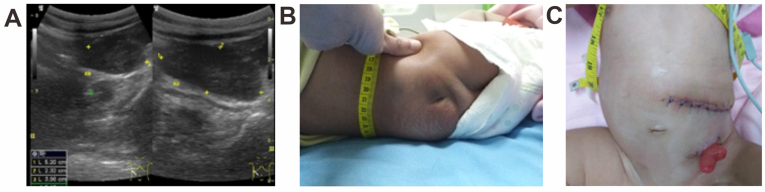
A) Abdominal ultrasound showed no abnormalities in the right kidney, but the left kidney was not visualized; B) Sacral dimple; and C) Stoma on the abdominal wall post-colostomy at 3 weeks of age (this image was taken after diaphragmatic hernia surgery).
